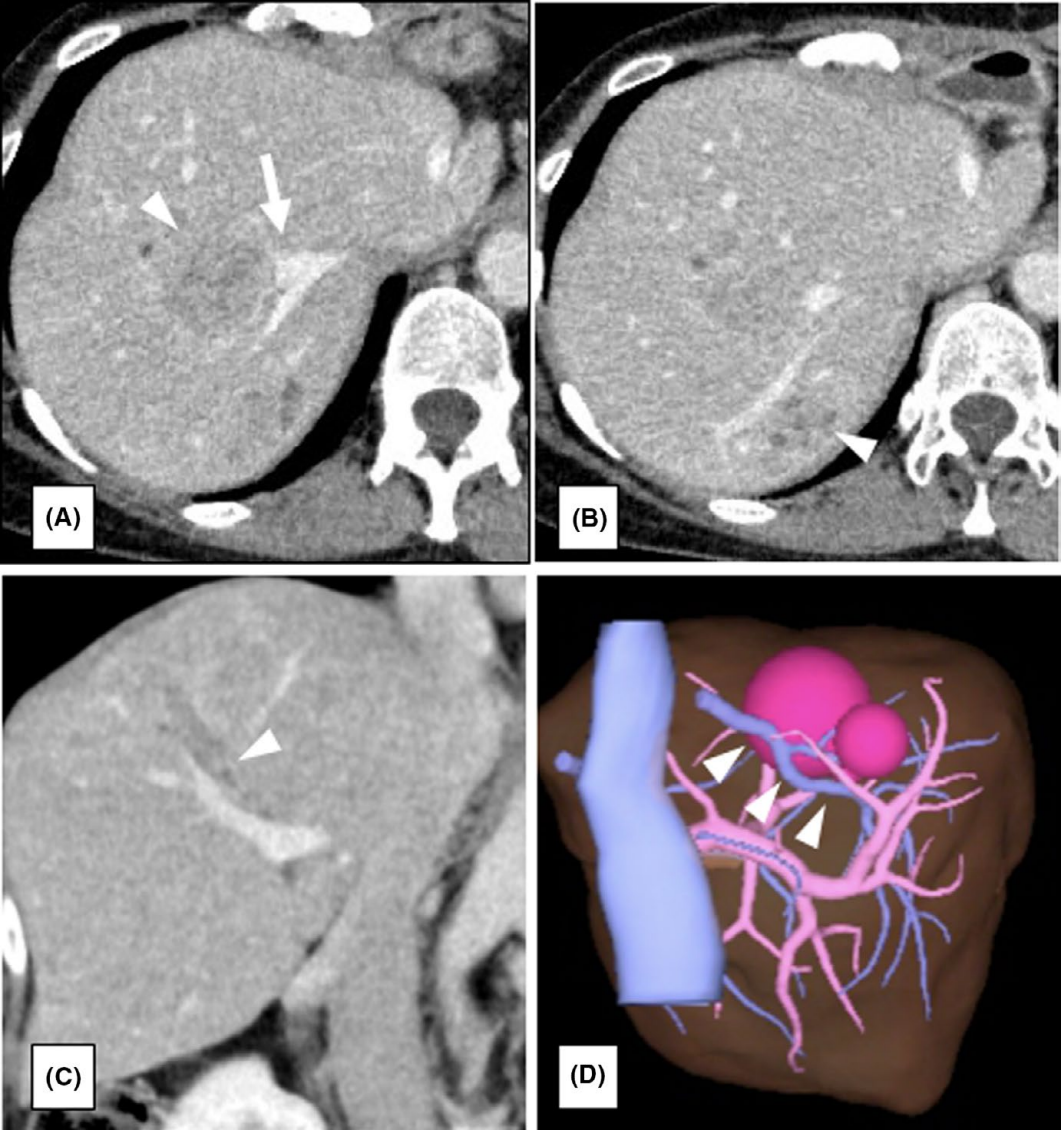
A, B, Contrast‐enhanced CT before portal vein embolization. Metastatic tumors located at segment 7 & 8 in the remnant liver (arrowhead). One of the metastatic tumor in segment 8 involved the right hepatic vein (arrow). C, Glissonean invasion of the main tumor in segment 8. The arrow head shows the glissonean invasion of the main tumor in segment 8. D, The 3D image of the liver. The main tumor in segment 8 involved the right hepatic vein for 4 cm in length (arrow head)
